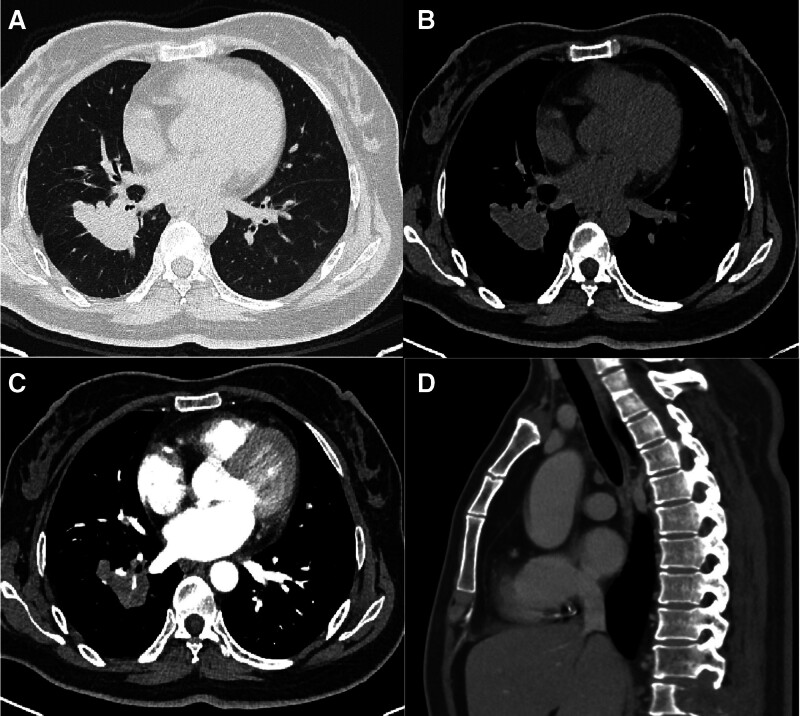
A 47-year-old woman who presented with low back pain for 3 months was diagnosed with adenocarcinoma in the right lower lobe. (A, B) A CT scan shows a soft-tissue mass in the right lower lung. (C) Enhanced CT demonstrates the uneven enhancement lesion surrounding the right lower lobe subsegmental pulmonary artery. (D) Sagittal images show bone destruction and soft-tissue involvement in the 11th thoracic vertebral.
